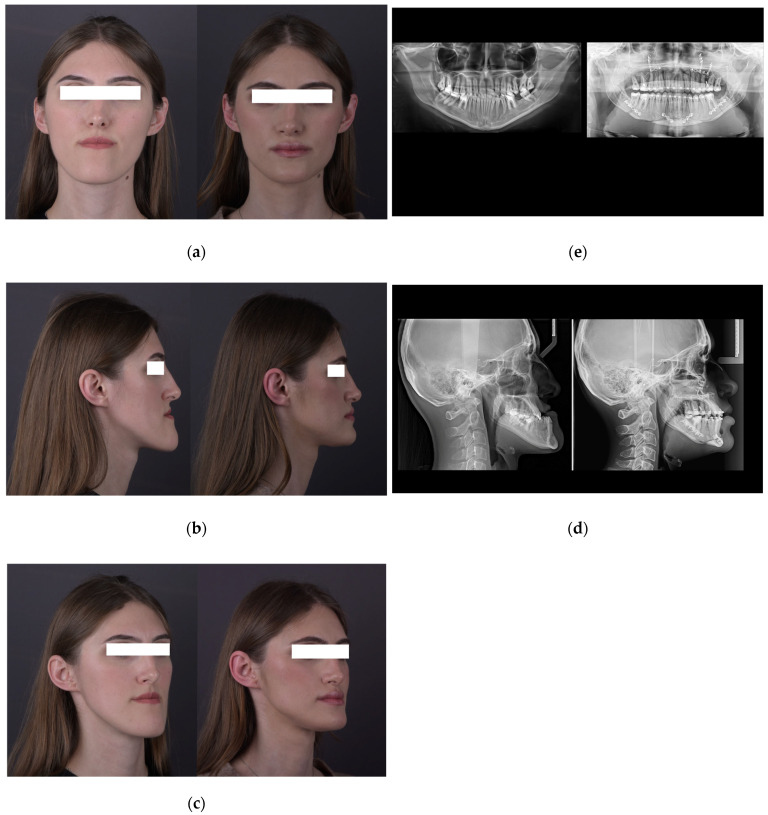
(a,e) Frontal facial view and panoramic X-rays, pre- and post-surgery: Improved midface projection, facial symmetry, and postoperative bone fixation. (b,d) Lateral profile and lateral cephalometric X-rays: Balanced facial profile after maxillary advancement and mandibular retrusion; fixation visible postoperatively. (c) Semi-profile view: Enhanced jawline contour and facial proportions following surgery.
